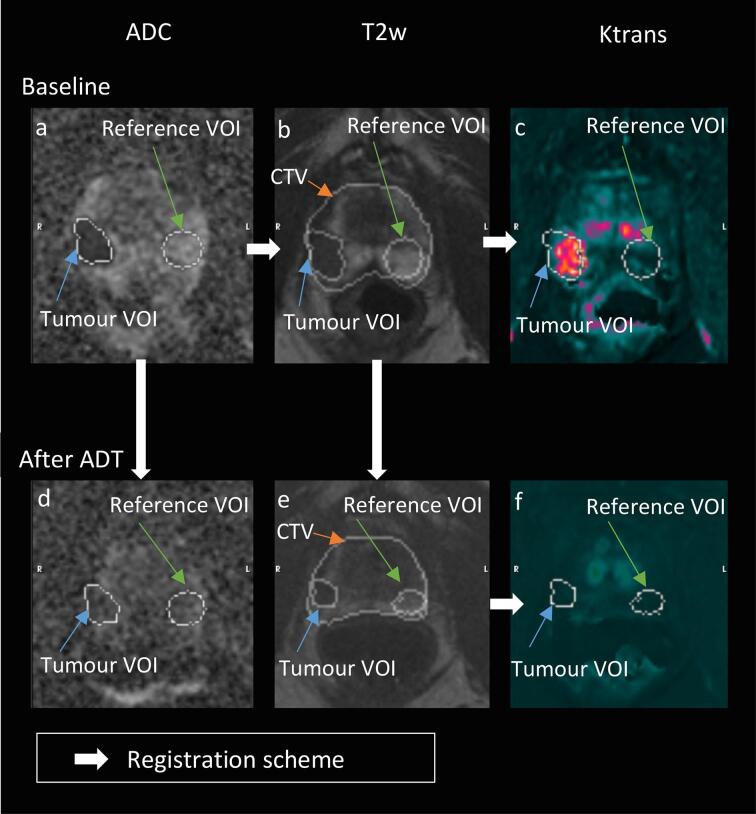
Multiparametric MRI (ADC, T2w and Ktrans from DCE MRI) from a patient in the study. a-c are baseline, and d-f are post-ADT. White arrows indicate registration order. PSA at baseline: 48 ng/ml, PSA post ADT: 0.1 ng/ml. a) ADC at baseline: Tumour VOI = 0.5 × 10^−3^ mm^2^/s, Reference VOI = 1.5 × 10^−3^ mm^2^/s. b) T2w at baseline, volumes: CTV = 55 cm^3^, Tumour VOI = 2.9 cm^3^, Reference VOI = 5.7 cm^3^. c) Ktrans at baseline: Tumour VOI = 0.11 min^−1^, Reference VOI = 0.02 min^−1^. d) ADC after ADT: Tumour VOI = 1.0 × 10^−3^ mm^2^/s, Reference VOI = 1.0 × 10^−3^ mm^2^/s. e) T2w after ADT, volumes: CTV = 35 cm^3^, Tumour VOI = 1.4 cm^3^, Reference VOI = 4.3 cm^3^. f) Ktrans after ADT: Tumour VOI = 0.02 min^−1^, Reference VOI = 0.03 min^−1^.
